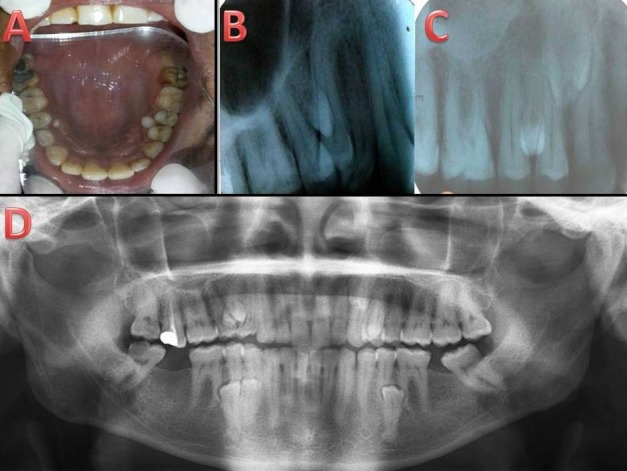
On extraoral examination in all five cases, there were no signs or symptoms of the presence of syndromic manifestations (Table 2). Based on clinical and radiographic findings, the cases were diagnosed as non-syndromic multiple erupted and impacted supernumerary teeth in relation to premolars (Table 1). Since the patients were completely asymptomatic, they were informed about supernumerary teeth and advised to be on regular follow-up.
